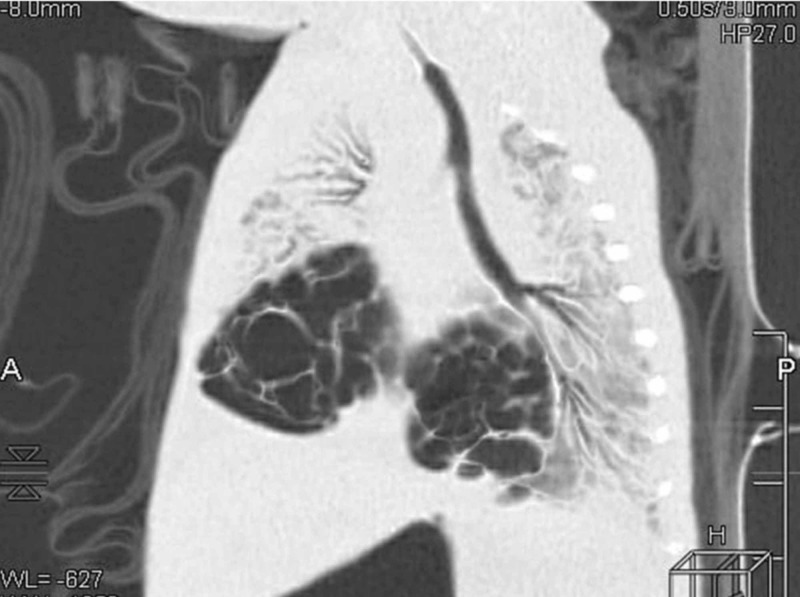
Chest computed tomography (CT), sagittal view, showing hyperinflation of the right middle lobe and atelectatic remaining lobes
